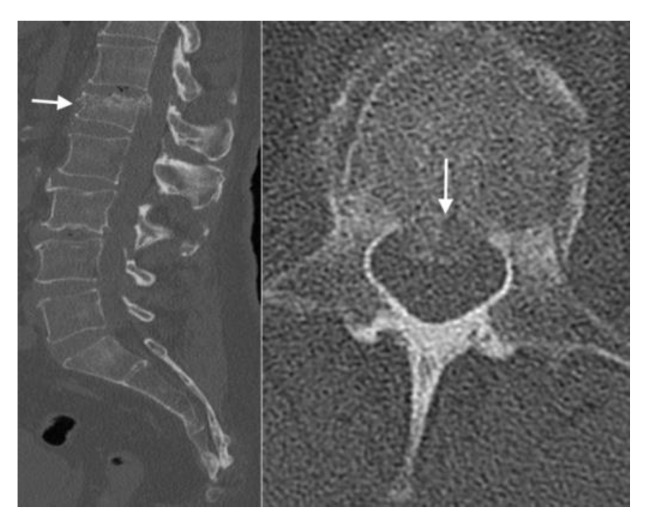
In this sagittal CT (left) of the spine, the arrow shows acute to subacute burst compression fracture of the superior endplate of L1 with approximately 40% height loss. The bones are diffusely demineralized. In this axial CT (right) of the L1 vertebral body, the arrow shows retropulsion of fragments with moderate spinal canal narrowing and flattening of the thecal sac.
